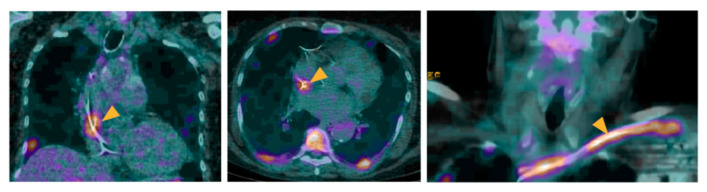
Role of ^18^F-FDG-PET/CT in a patient with a suspicion of IE on CIED.
